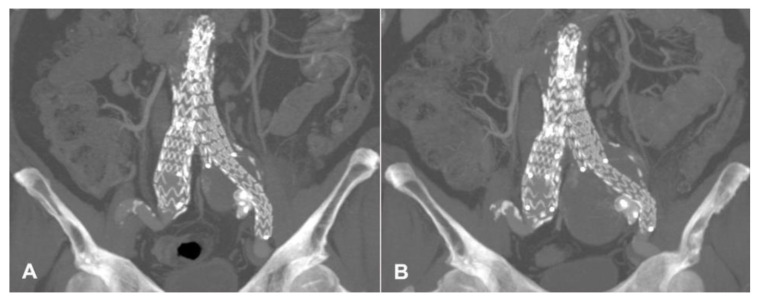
Enhanced computed tomography scan showing the left common iliac artery aneurysm after coil embolization and external iliac extension (A) and its enlargement with late contrast phase endoleak at 3-year follow-up (B).
